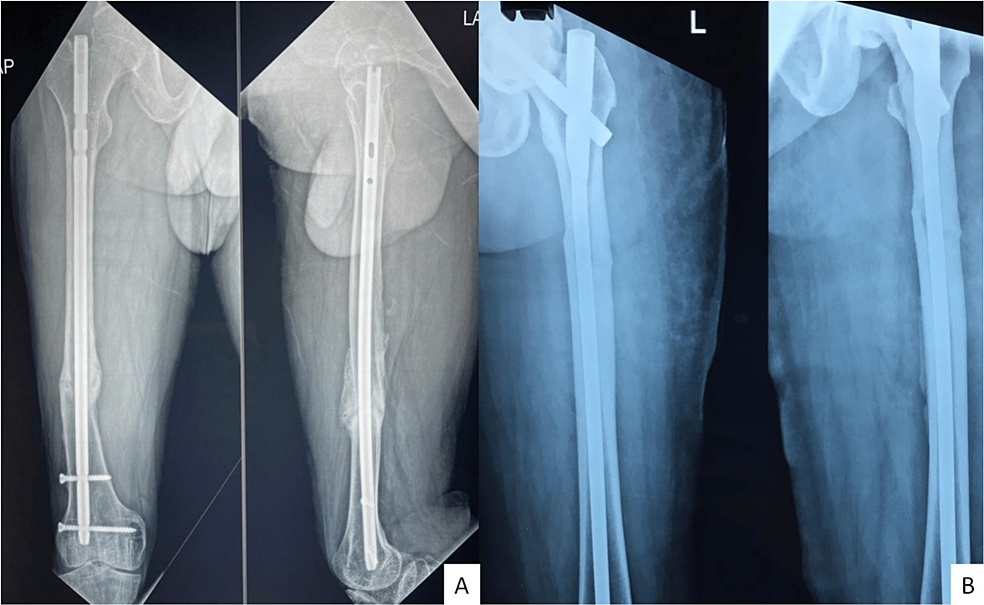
One-year postoperative X-ray of the healed fractures of patients 2 and 9.
